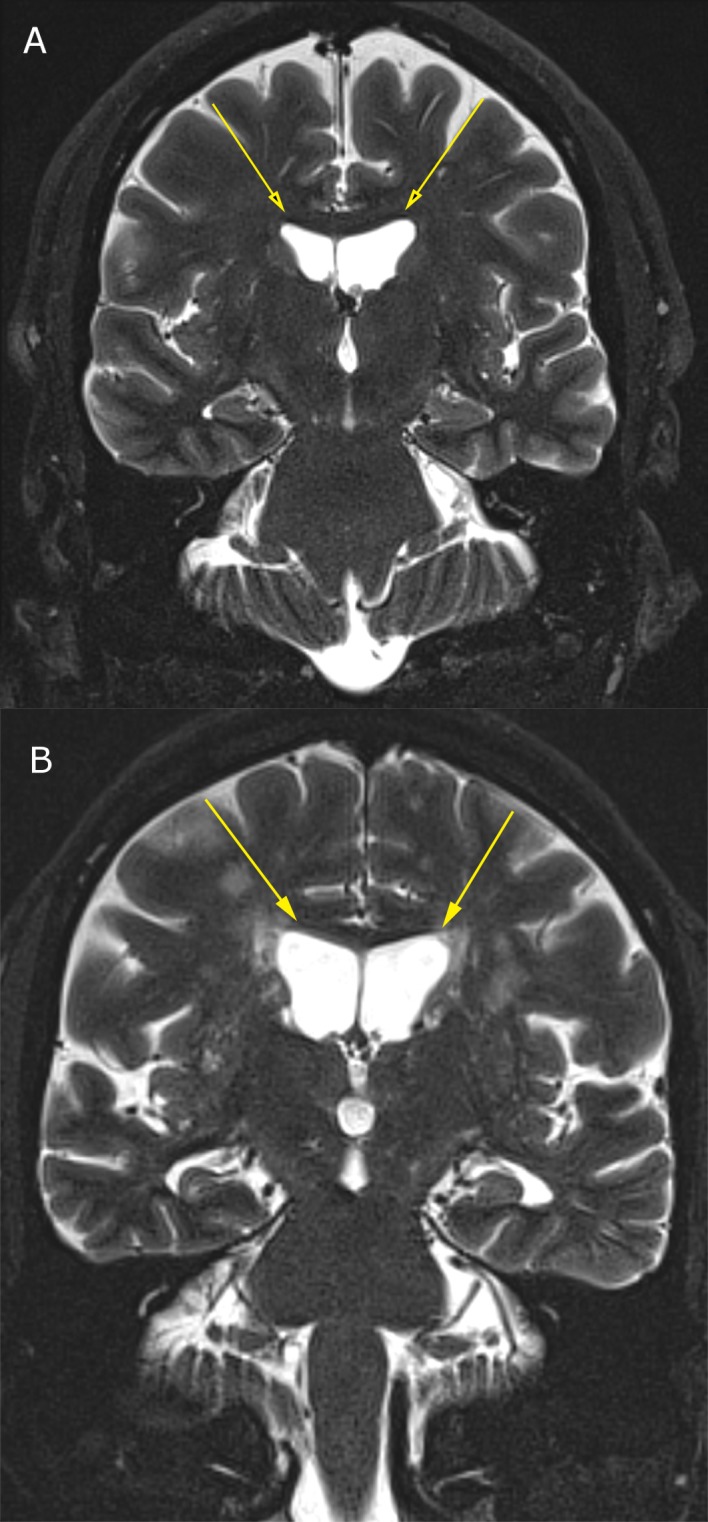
3T MRI of lateral ventricular enlargement due to central atrophy; (3a) Normal lateral ventricular size in 55-year-old male; (3b) Moderately enlarged lateral ventricles due to central atrophy in 68-year-old male.
